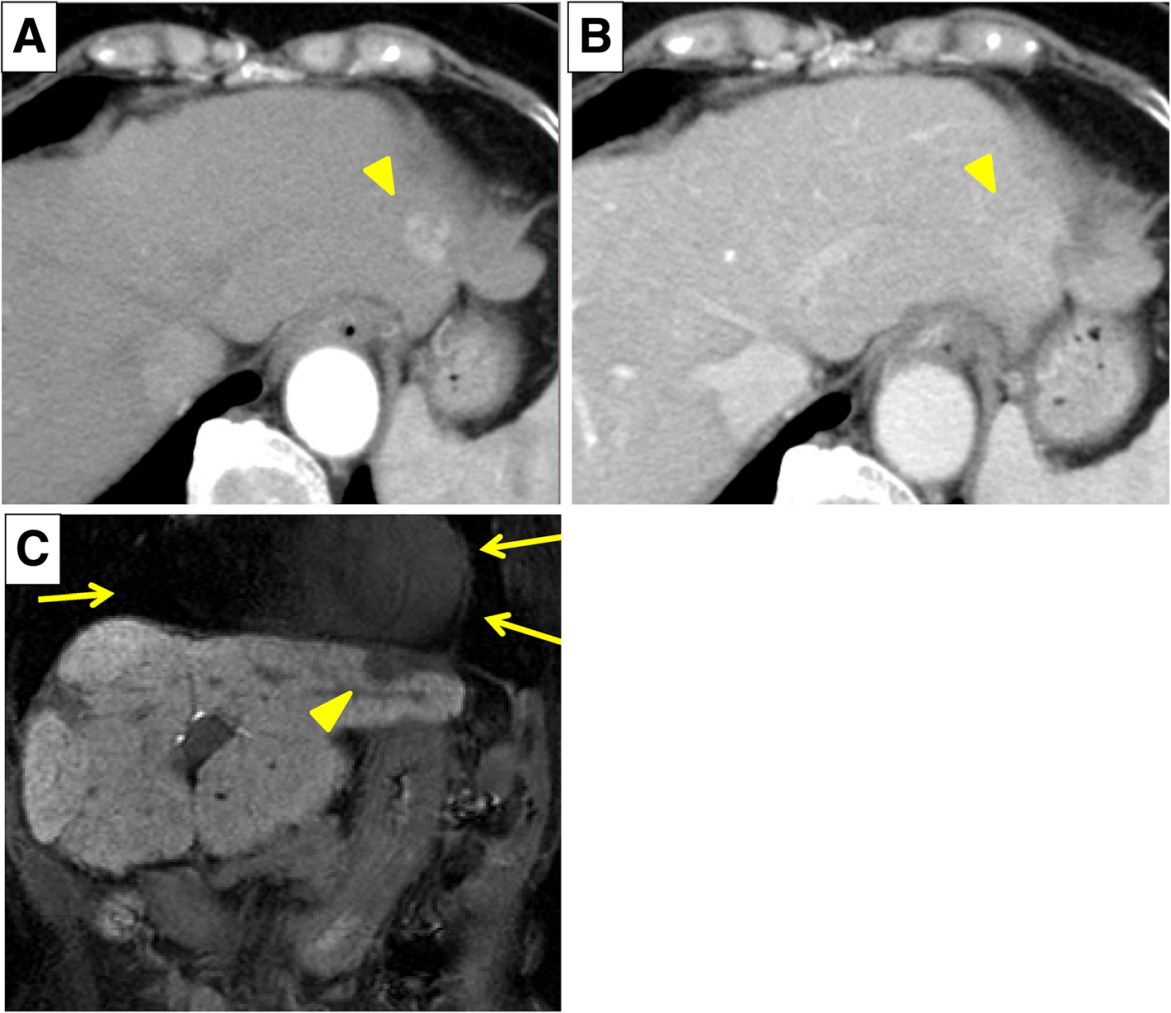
Abdominal enhanced computed tomography prior to laparoscopic RFA. There was a 1.5-cm mass in the left lateral lobe of the liver (arrow head). a arterial phase, b portal phase, and c ethoxybenzyl diethylenetriamine-enhanced magnetic resonance imaging prior to laparoscopic RFA. The tumor (arrow head) was located just below the heart (arrows)
